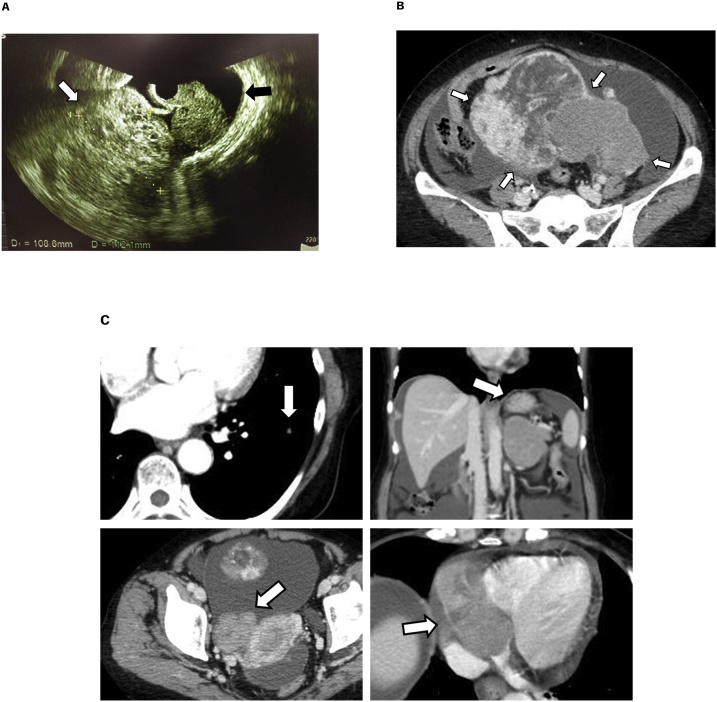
A, Pelvic ultrasonography showed an 11-cm solid mass in the pelvis that was rough, hard, and had poor mobility (white arrow). In addition, abundant ascites was also identified (black arrow). B, Computed tomography (CT) revealed a huge ovarian mass at left adnexa that occupied the pelvic cavity. C, CT also identified multiple metastases that included the lung (upper left), adrenal gland (upper right), right adnexa (lower left), and heart (lower right) (white arrow).
